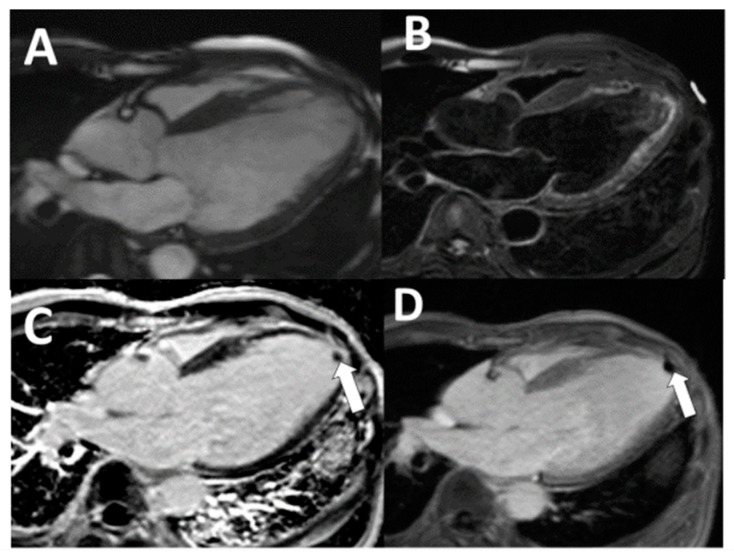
Example of false-negative cine-CMR and DB-CMR. Echocardiography was interpreted as negative (not shown). LV apical thrombus was not detected on (A) cine-CMR and (B) DB-CMR. LV apical thrombus was demonstrated on standard LGE-CMR with an inversion time of (C: arrow) 250–350 ms and (D: arrow) long inversion time with inversion time = 600 ms. This patient subsequently obtained antithrombotic medication after CMR. (CMR: Cardiac magnetic resonance imaging, LGE-CMR: Late gadolinium enhancement sequence, DB-CMR: Dark blood sequence).
